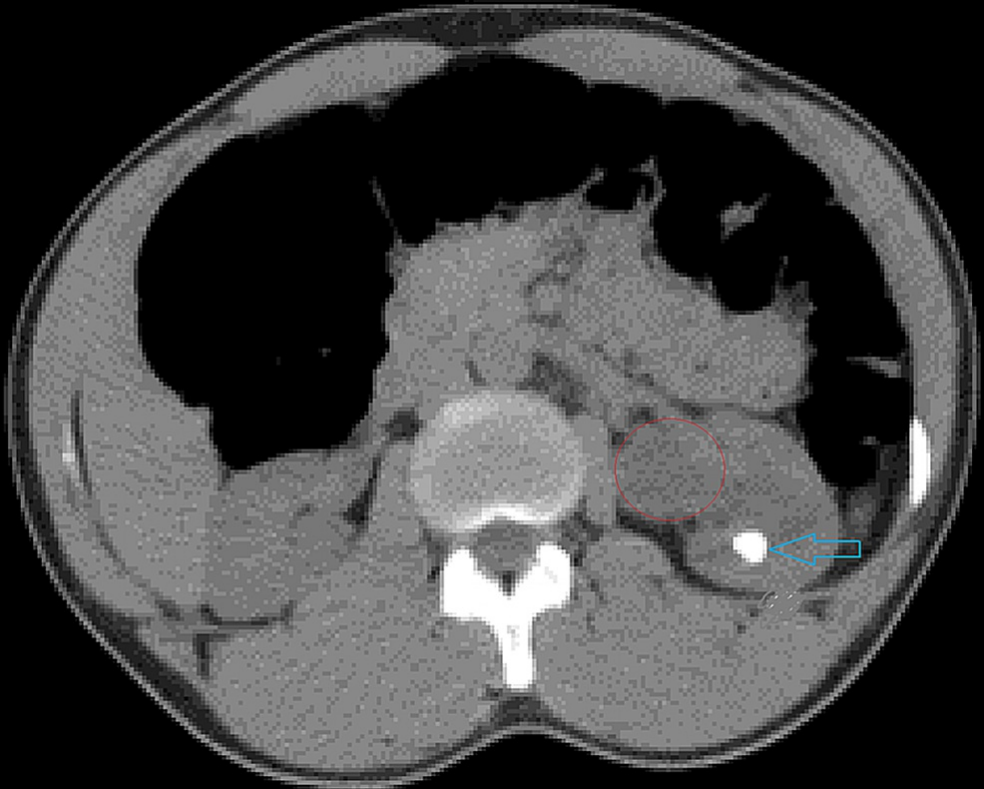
Non-contrasted CT scan showing left-sided 12.7 mm x 9.8 mm renal calculus (blue arrow) and dilated uretero-pelvic junction segment (red circle).
